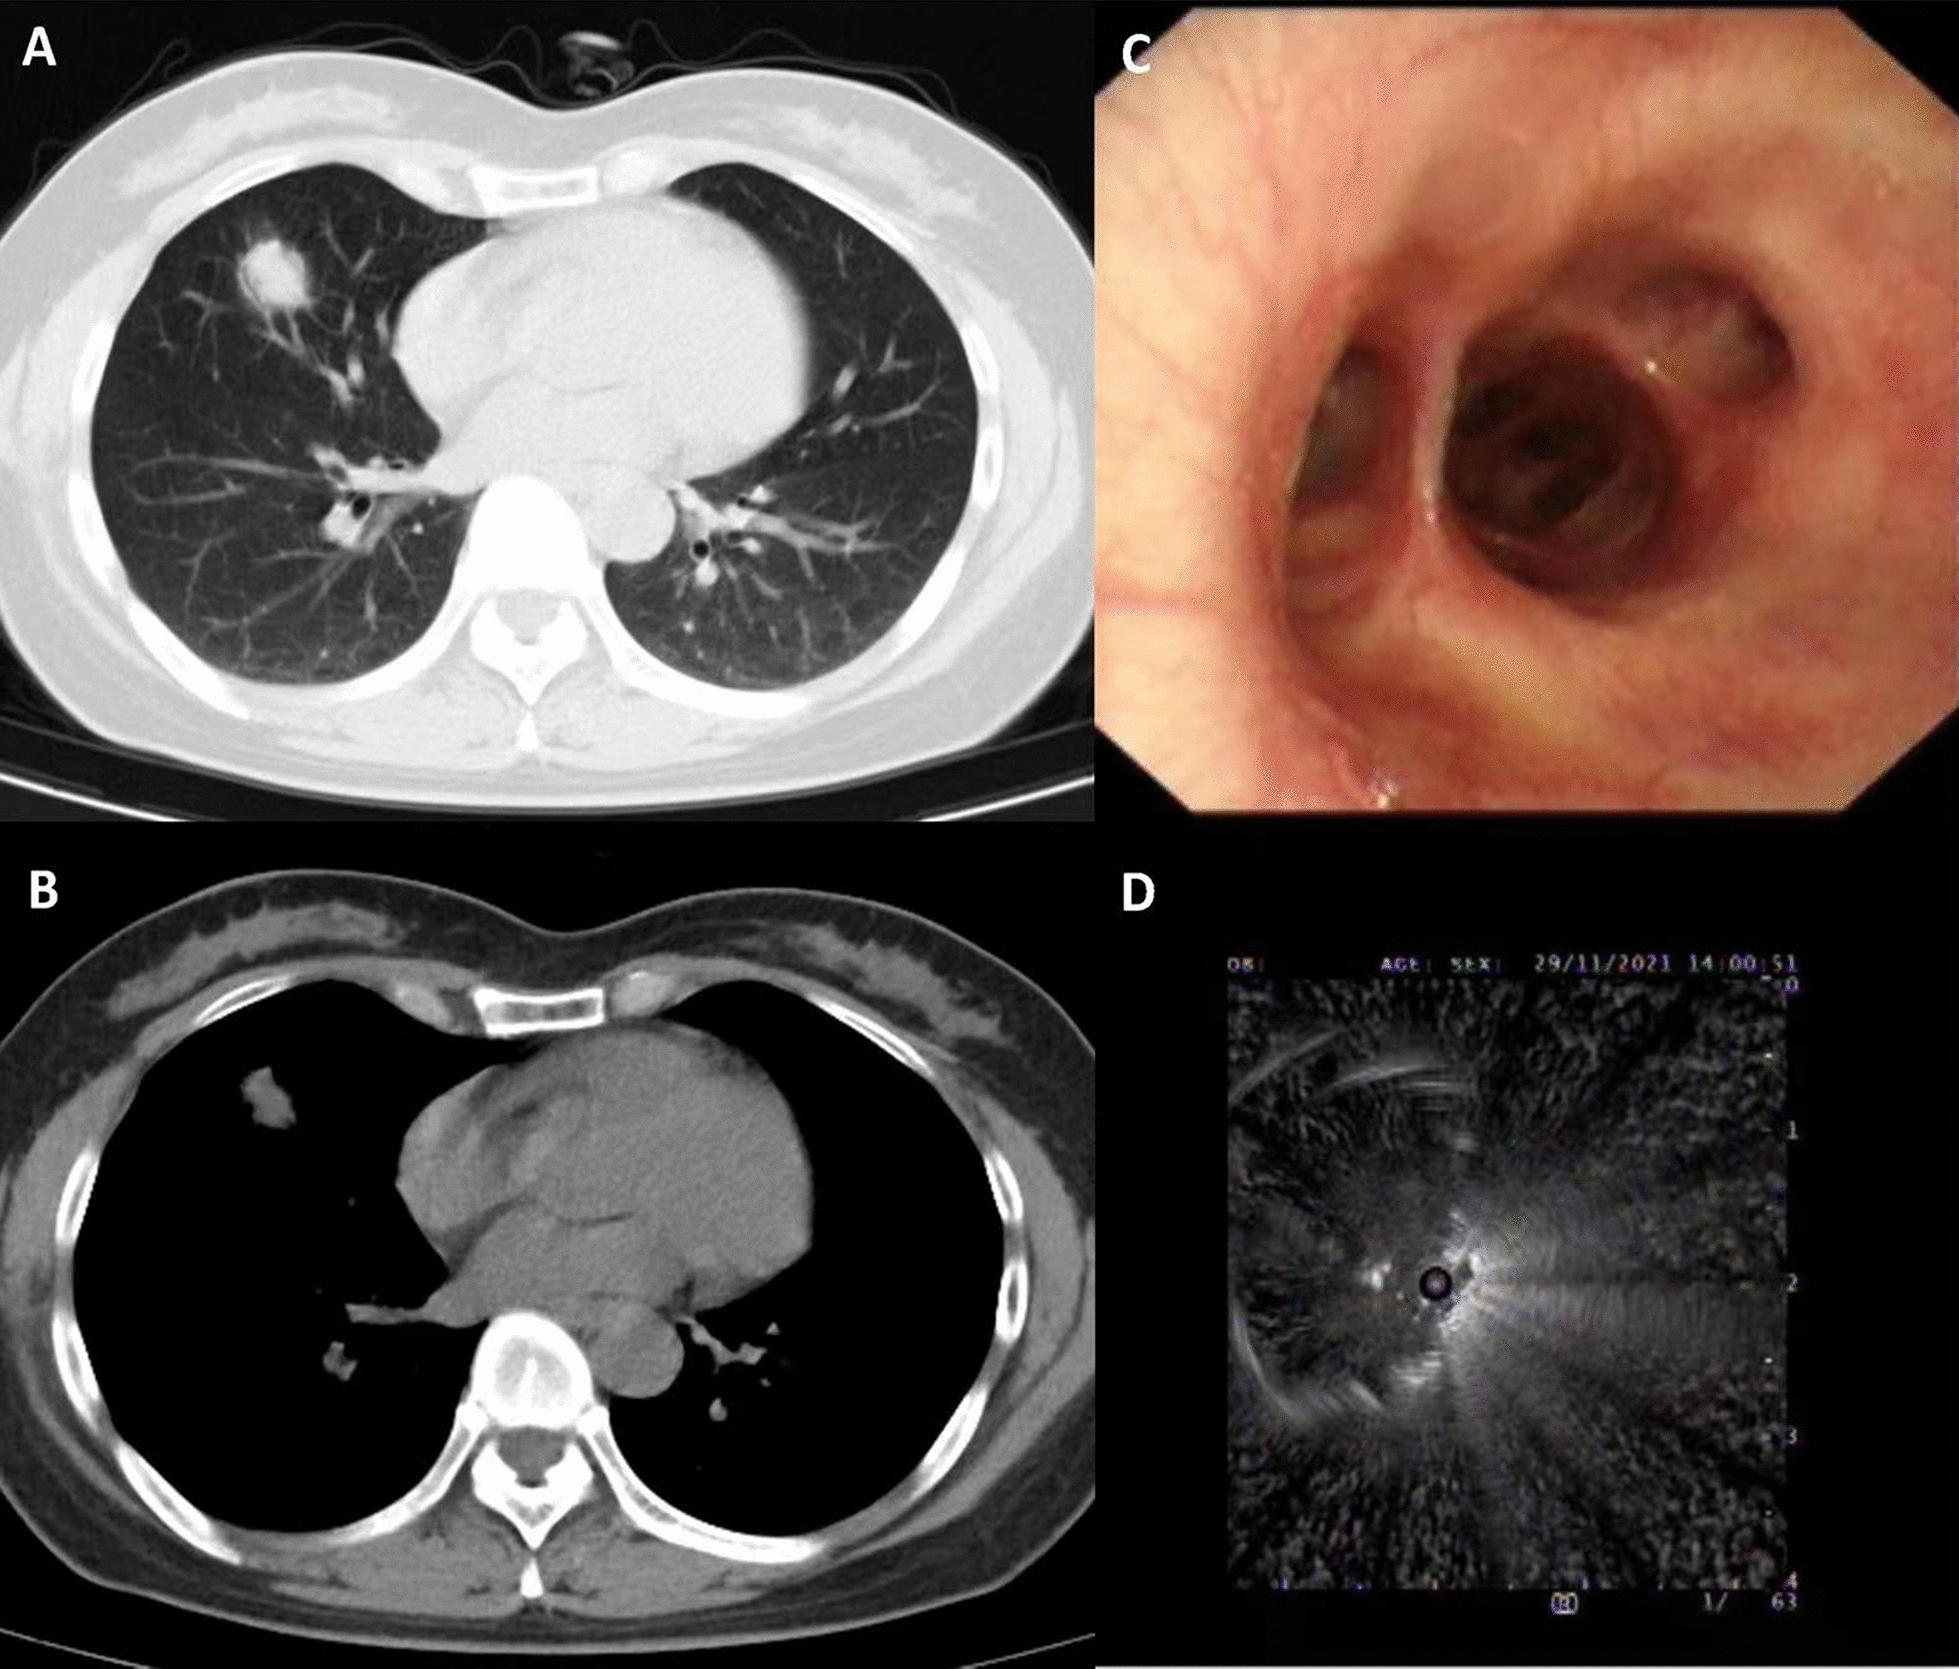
Case 1, a 45-year-old female who was diagnosed with pulmonary tuberculosis in the middle lobe of the right lung. A, B Chest CT, showing of lesions in the lung window and mediastinal window. C, D Ultrasound from EBUS-GS, showing lesions around the ultrasound probe
